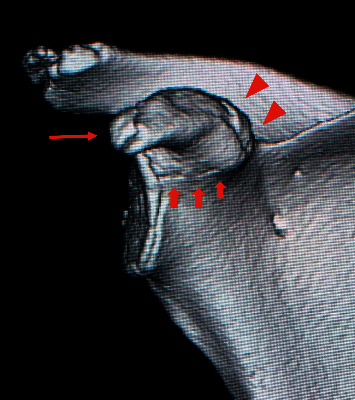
Representative three-dimensional computed tomography image of a normal epiphyseal line around the coracoid of an 11-year-old girl. A normal coronoid process has three epiphyseal lines: the base (broad arrows), center (triangular arrows), and tip (narrow arrow).
